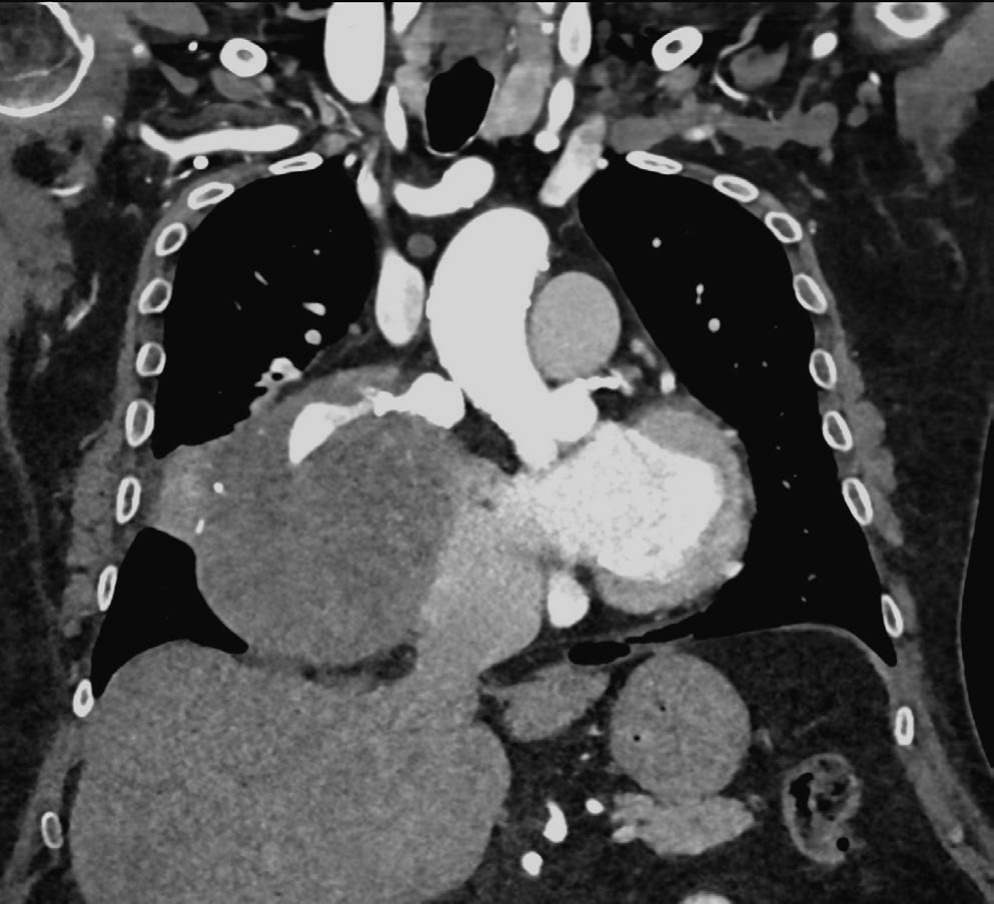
CTA, coronal plane, arterial phase. The communication with the right coronary artery (RCA) proximal tract can be noticed.
